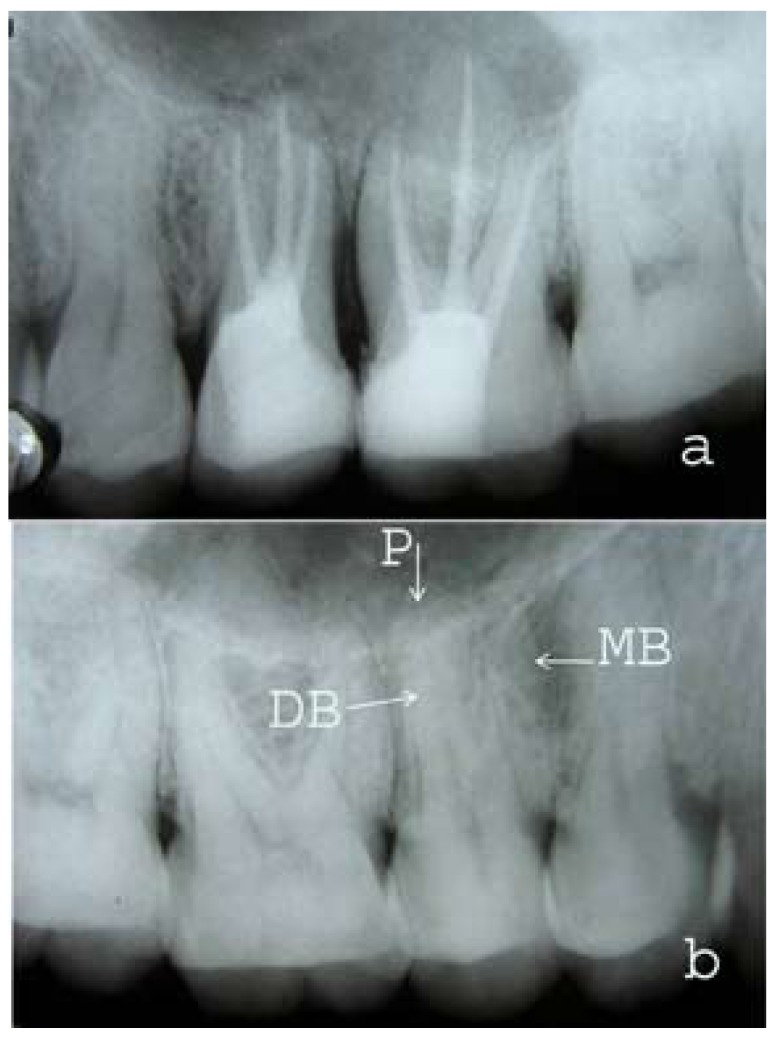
(a) Post-operative radiograph with all the three canals obturated in second premolar. (b) Radiograph showing the presence of three-rooted maxillary right second premolar in the same patient (arrows pointing; P-Palatal, MB-Mesiobuccal, DB-Distobuccal).
